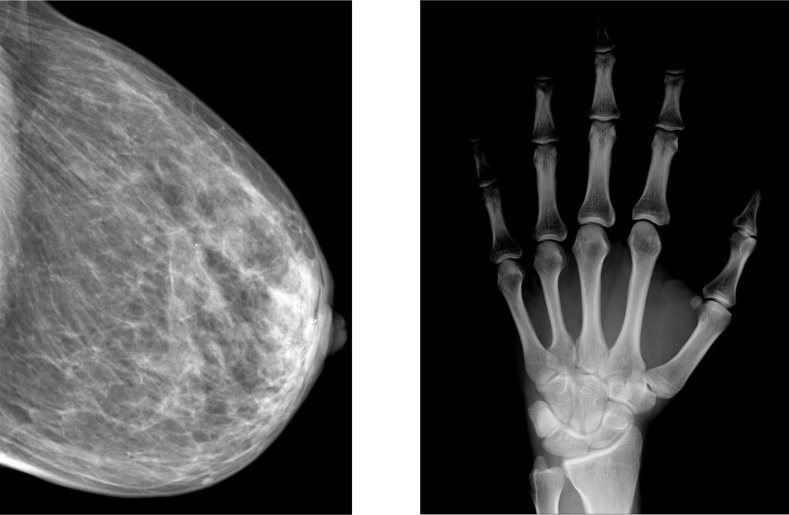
Two typical x-ray images from an a-Se FPXI. Left, a typical x-ray image of a breast. Right, an x-ray image of a hand.
